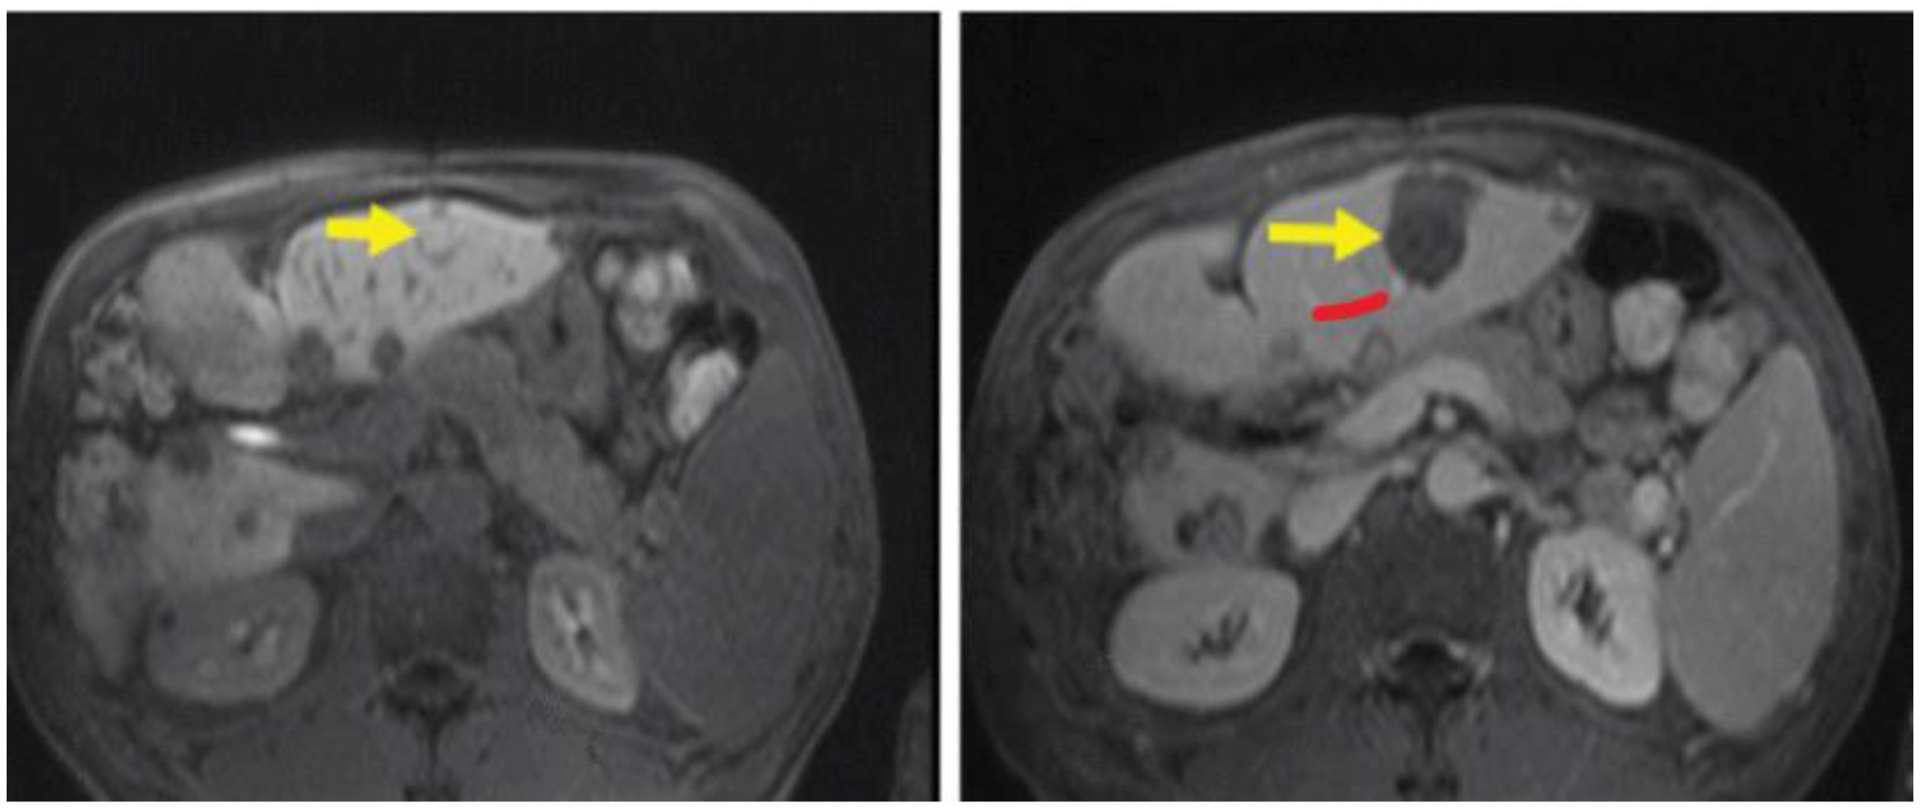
Posthistotripsy contrast-enhanced T1w MRI. MRI of the targeted tumor 5 mm adjacent to hepatic vessel branch (red arrow) before (a) and after (b) histotripsy procedure (yellow arrow). Figure adapted from [10].
